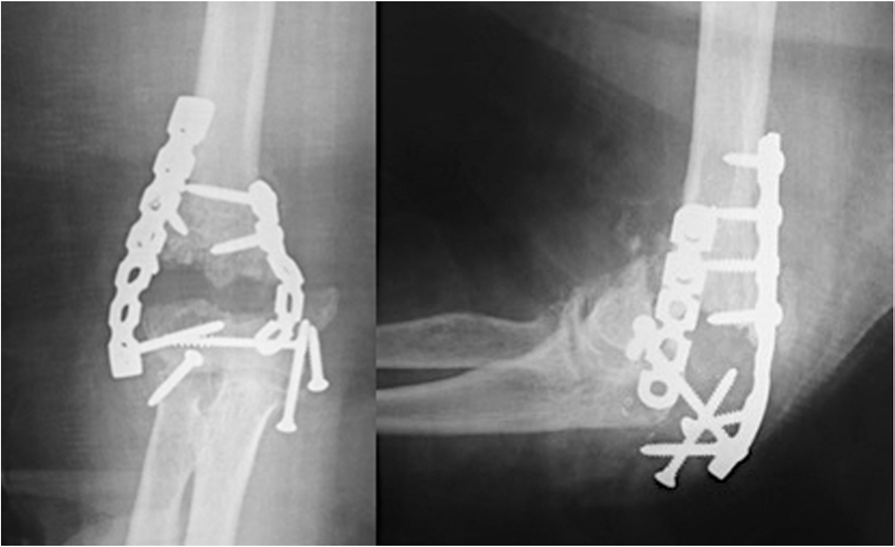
X-rays at presentation of a 69 years’ old lady (case #9) with low-laying fracture of the distal humerus that have failed a previous osteosynthesis
